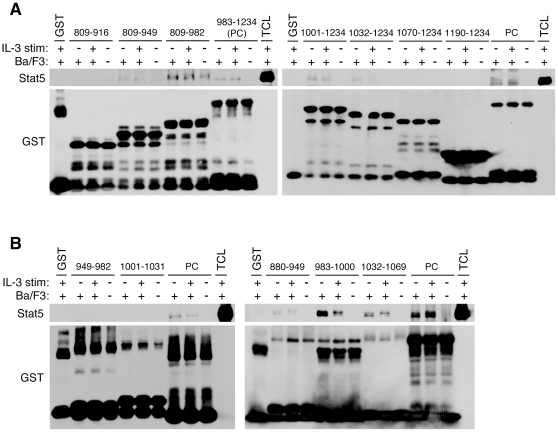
Mapping the Stat5-binding sites within PLC-β3-CT. (A, B) Lysates of Ba/F3 cells incubated with or without IL-3 were mixed with GST fusion proteins, then beads-bound proteins were analyzed by SDS-PAGE followed by immnoblotting with anti-Stat5 antibody. The same blots were reprobed with anti-GST. PLC-β3 portions of GST fusion proteins used are shown by residue numbers. TCL, total cell lysates. The 983–1234 fusion protein (PC) was used as a positive control while GST was used as a negative control.
